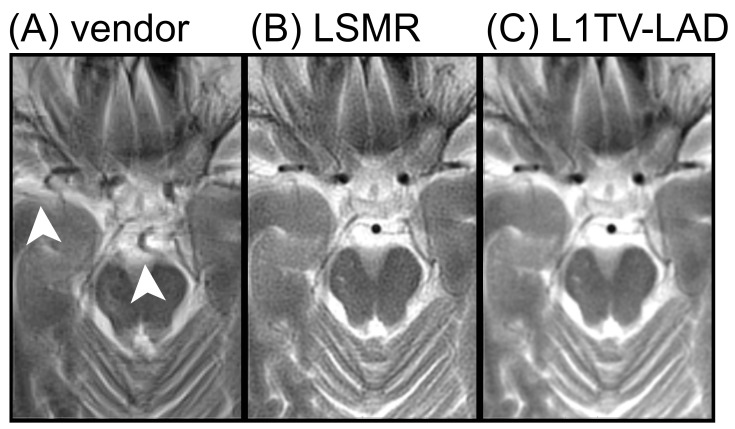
BLADE MRI with cooperative patients. (A) Erroneous correction for motion in the vendor-provided reconstruction, where the basilar artery is distorted and brain structures are overshadowed by artifacts (arrow heads). (B) Least-squares reconstruction without correcting motion. (C) L1TV-LAD reconstruction without correcting motion shows smooth imagery with a lower level of noise.
